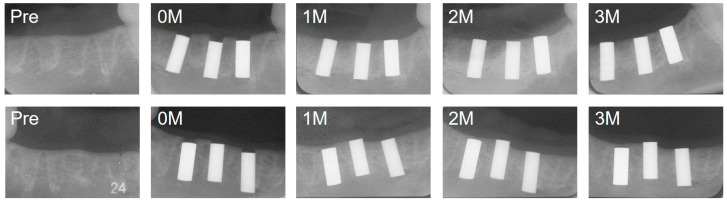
Serial radiographs of implant placement sites. Images were obtained before implantation (Pre), immediately after implantation (0M), and at 1, 2, and 3 months post-implantation. Upper row (left to right): Ti, 5Cu, and 20Ag; lower row (left to right): 30Ag, 10Cu, and 6Al4V.
